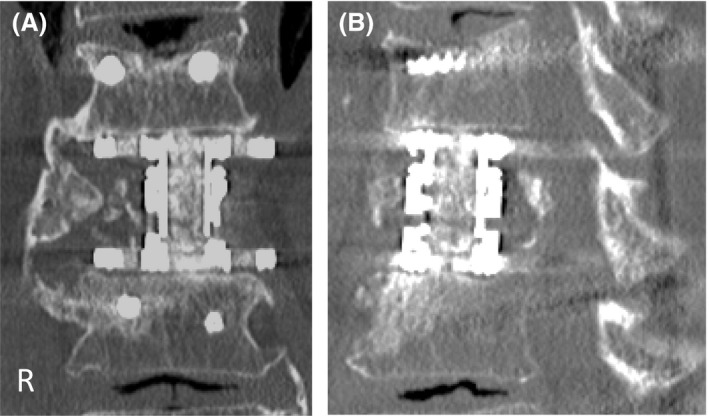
Computed tomography at 9 months after surgery. (A) Coronal and (B) sagittal sections of computed tomography images at 9 months after surgery. These showed continuity of trabecular bone between cage and endplate without implant failure or cage subsidence.
